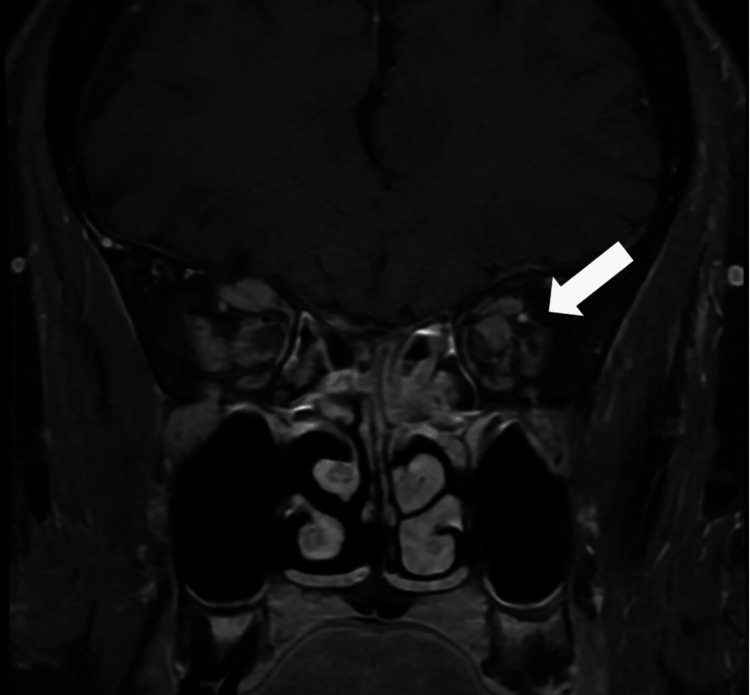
Orbital MRI Orbital MRI showing enlargement of both orbital nerves, with the superior branch of the oculomotor nerve compressing the left orbit nerve (arrow). MRI: magnetic resonance imaging
